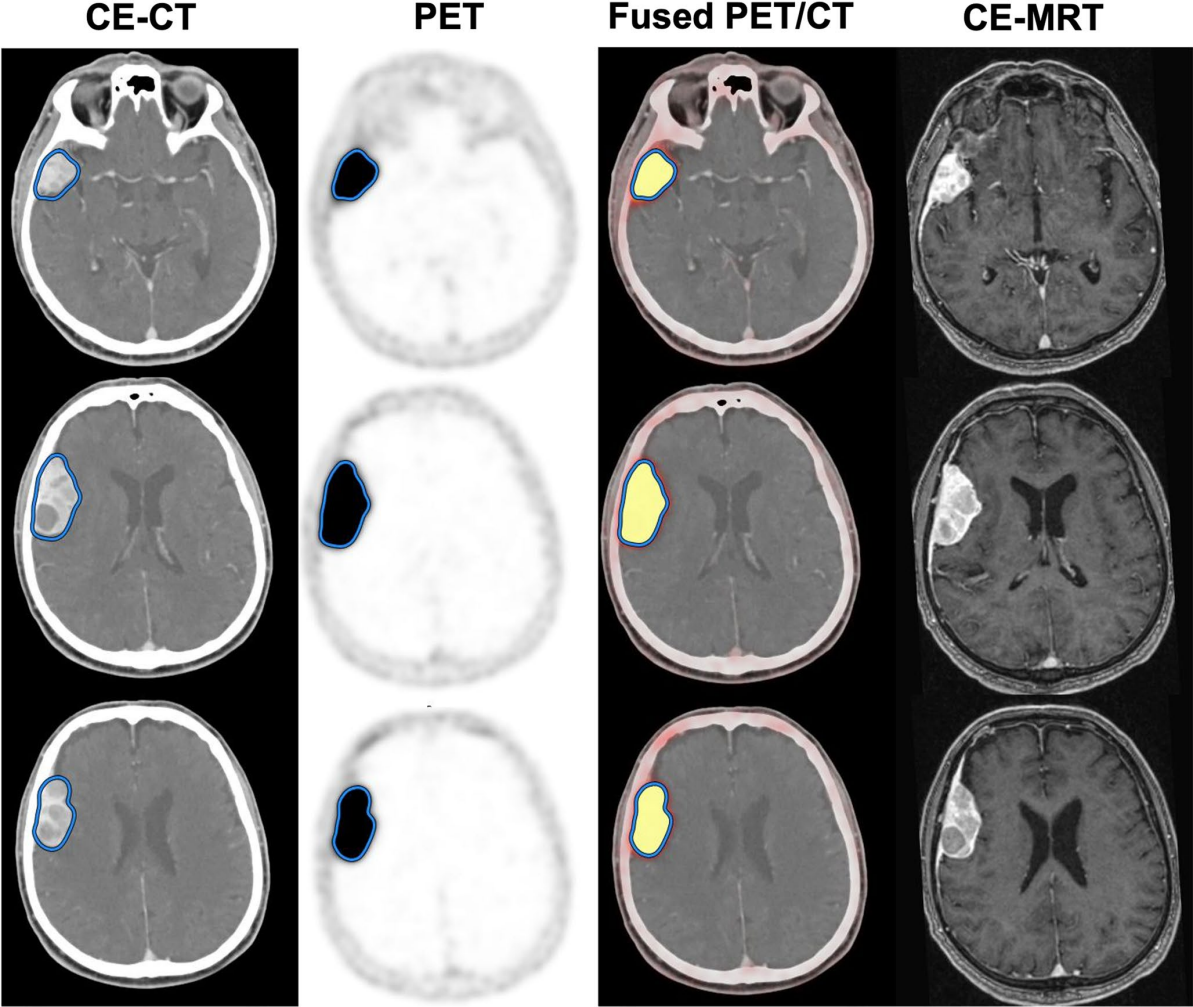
Example of SUV-thresholding for an extraosseous meningioma located in the right temporal region, prior to any treatment. The images are displayed in four columns, showing different modalities at the same axial level. From left to right: CE-CT, PET scan, fused PET/CT image, and corresponding CE-MRI on the far right
